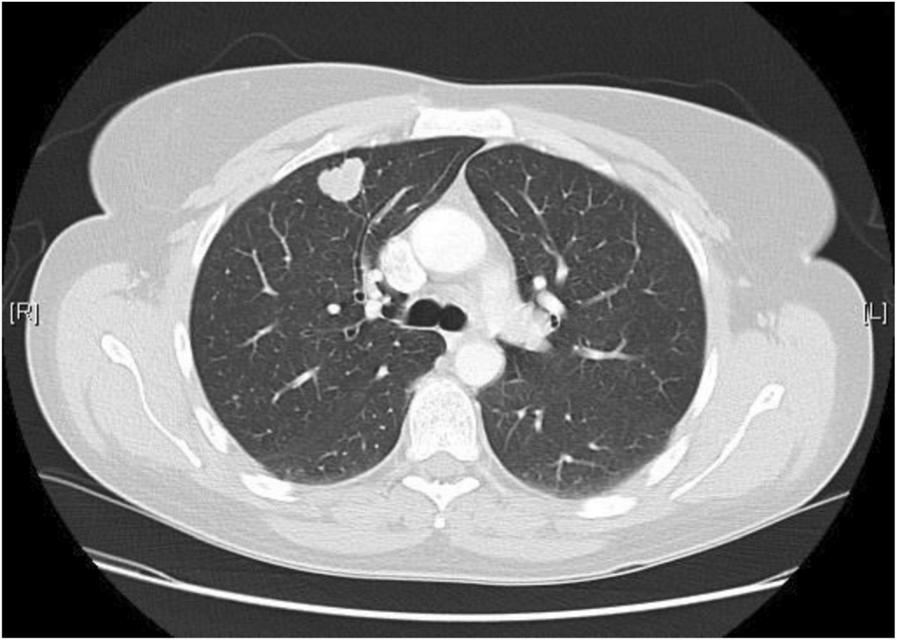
Computed tomography of the patient’s chest showing a speculated nodule in the upper field of her right lung
